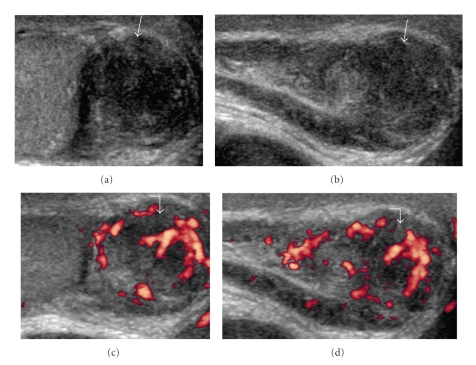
(a) (transverse), (b) (longitudinal) Grey-scale ultrasound images showing hypoechoic roundish mass (1.5 × 1.5 × 1.8 cm) in the tail of the right epididymis (white arrows). *Note*. The moderate vascularity on Power Doppler (c) (transverse), (d) (longitudinal) ultrasound (white curved arrows).
